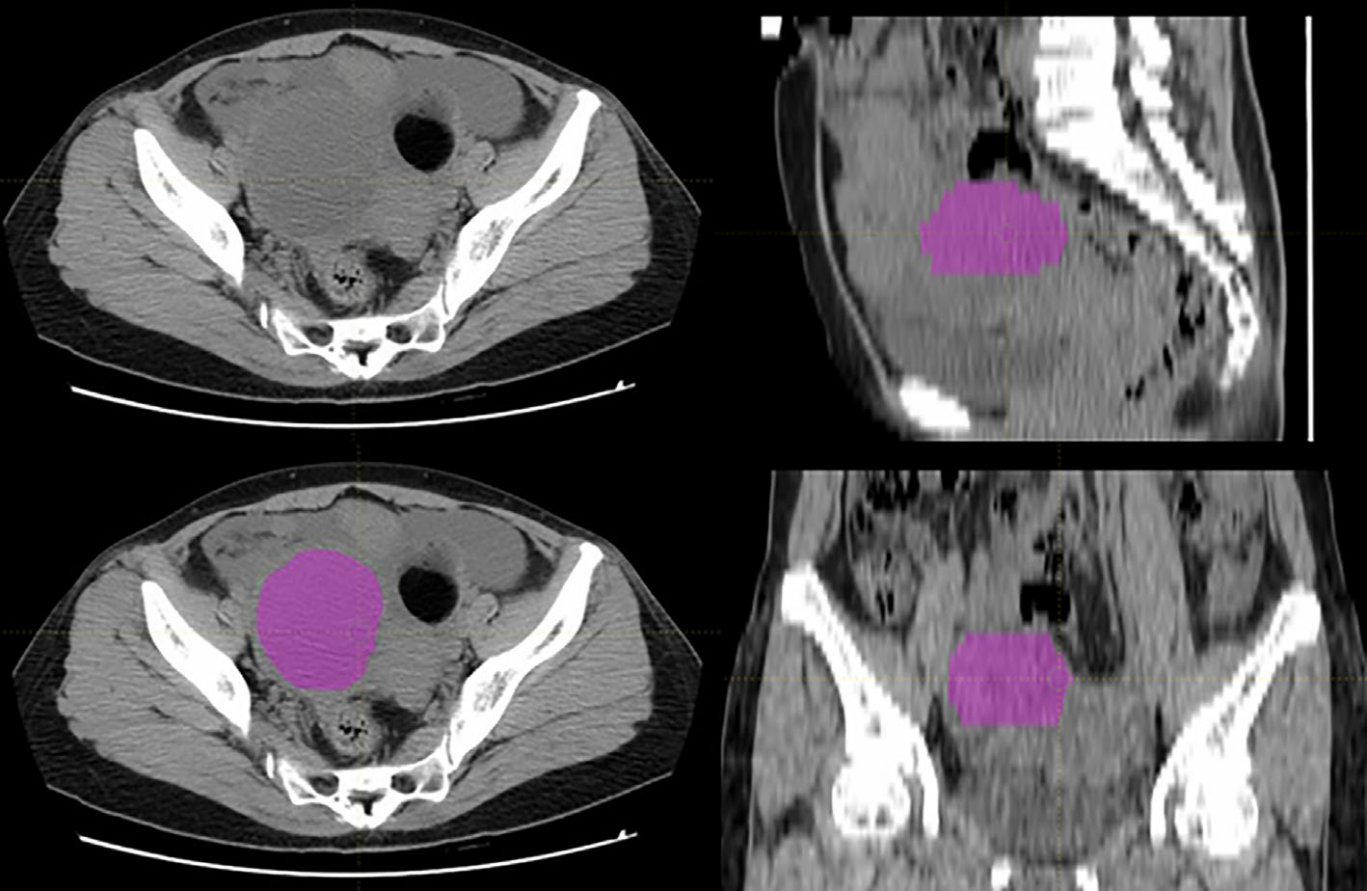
A typical ovarian tumor segmentation on CT images.
